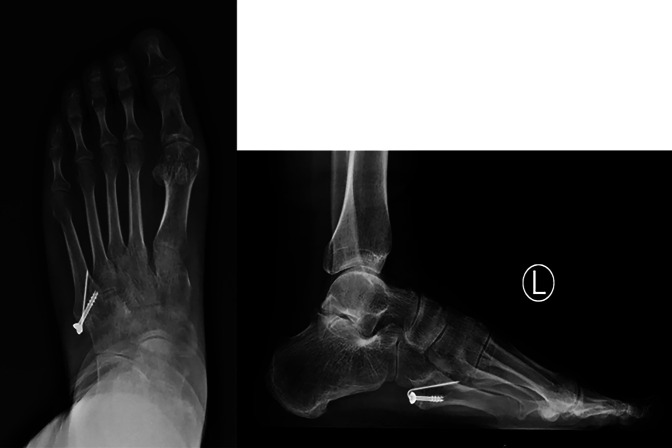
Postoperative 2 years radiographs.
